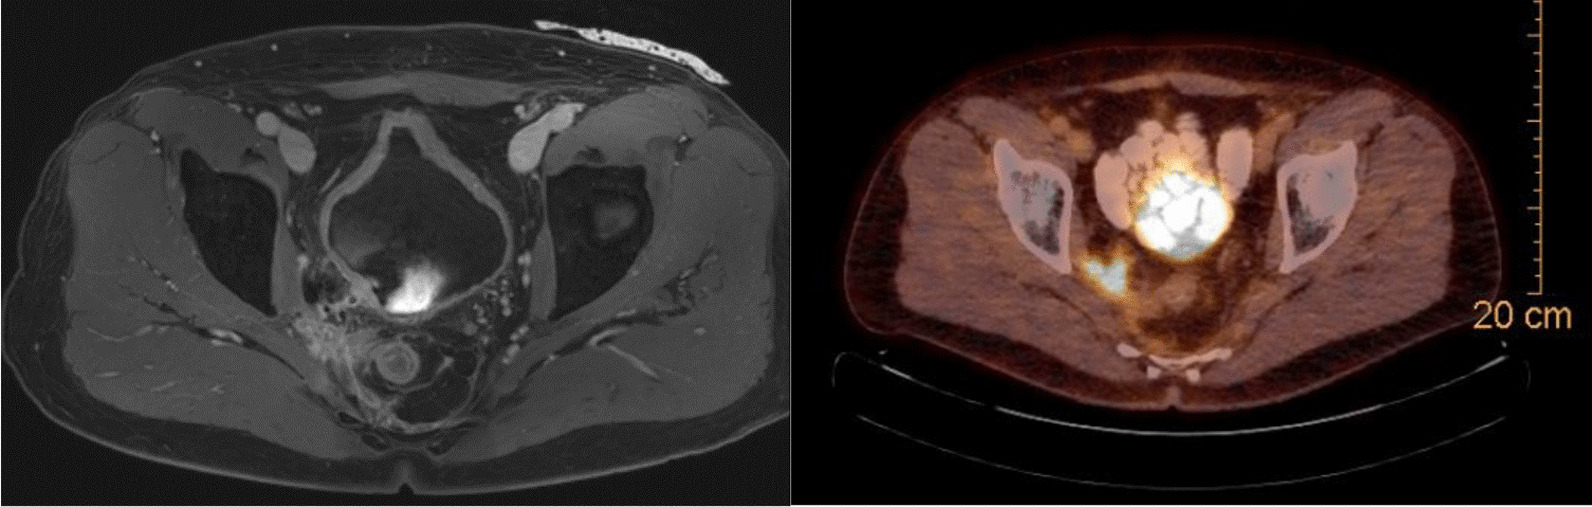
T1-weighted MRI (left) and FGD-PET CT with pelvic side wall recurrence prior to re-irradiation (right)
